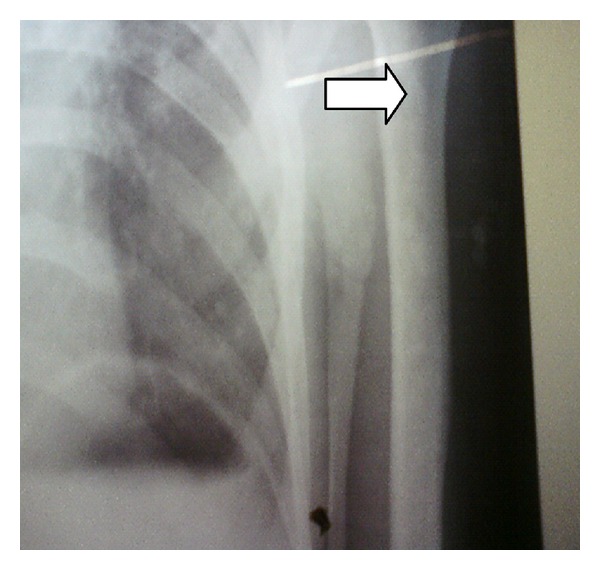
Chest radiograph showing osteosclerotic metastatic lesions in the upper end of humerus (white arrow).
